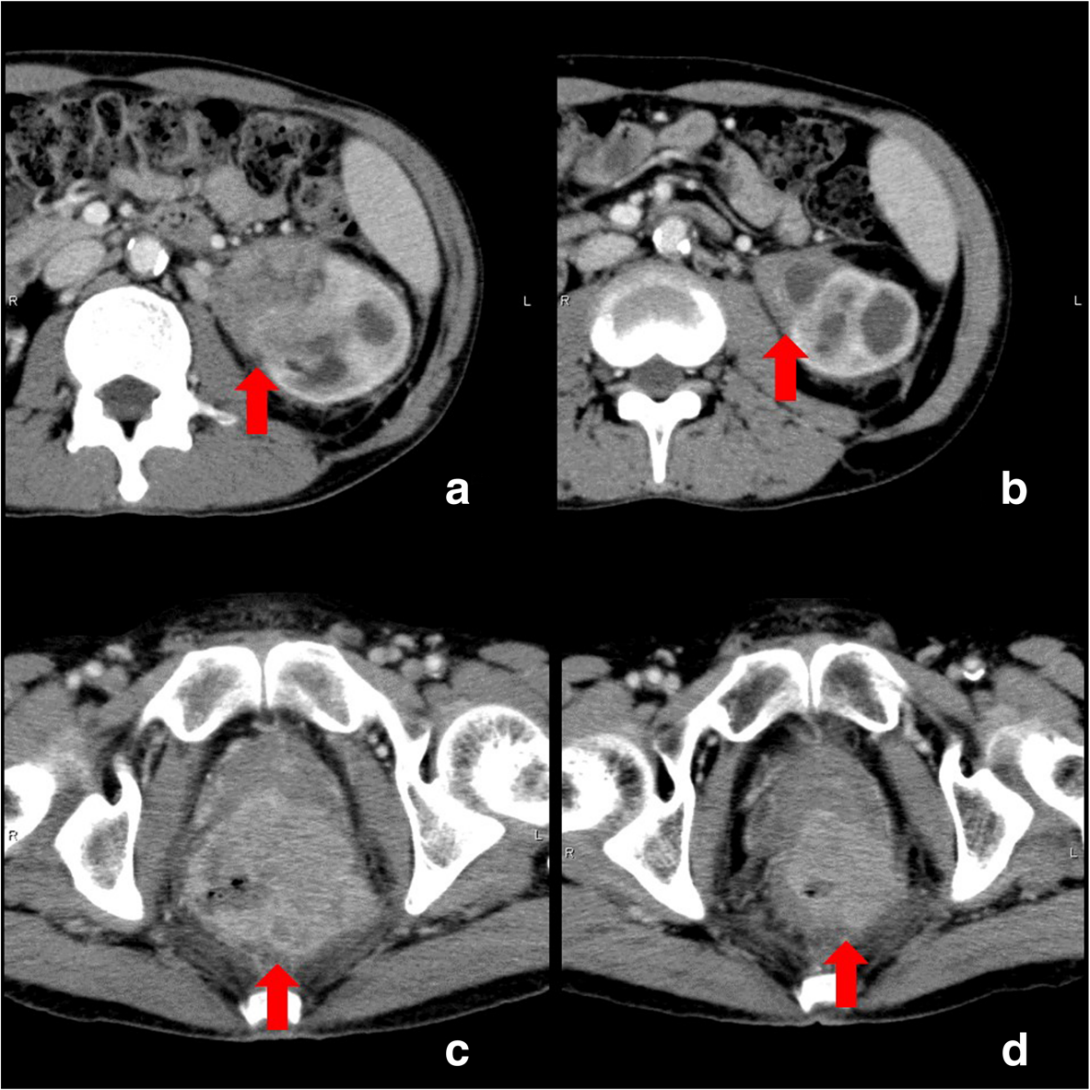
a, b Abdominal computed tomography scan demonstrating the volume of renal pelvic tumor before treatment (a), and the decrease in volume after two cycles of fluorouracil, leucovorin, oxaliplatin, and irinotecan (b). c, d The rectal cancer before treatment (c), and decrease in tumor volume after two rounds of fluorouracil, leucovorin, oxaliplatin, and irinotecan (d). *Red arrows* indicate the sites of the tumor
